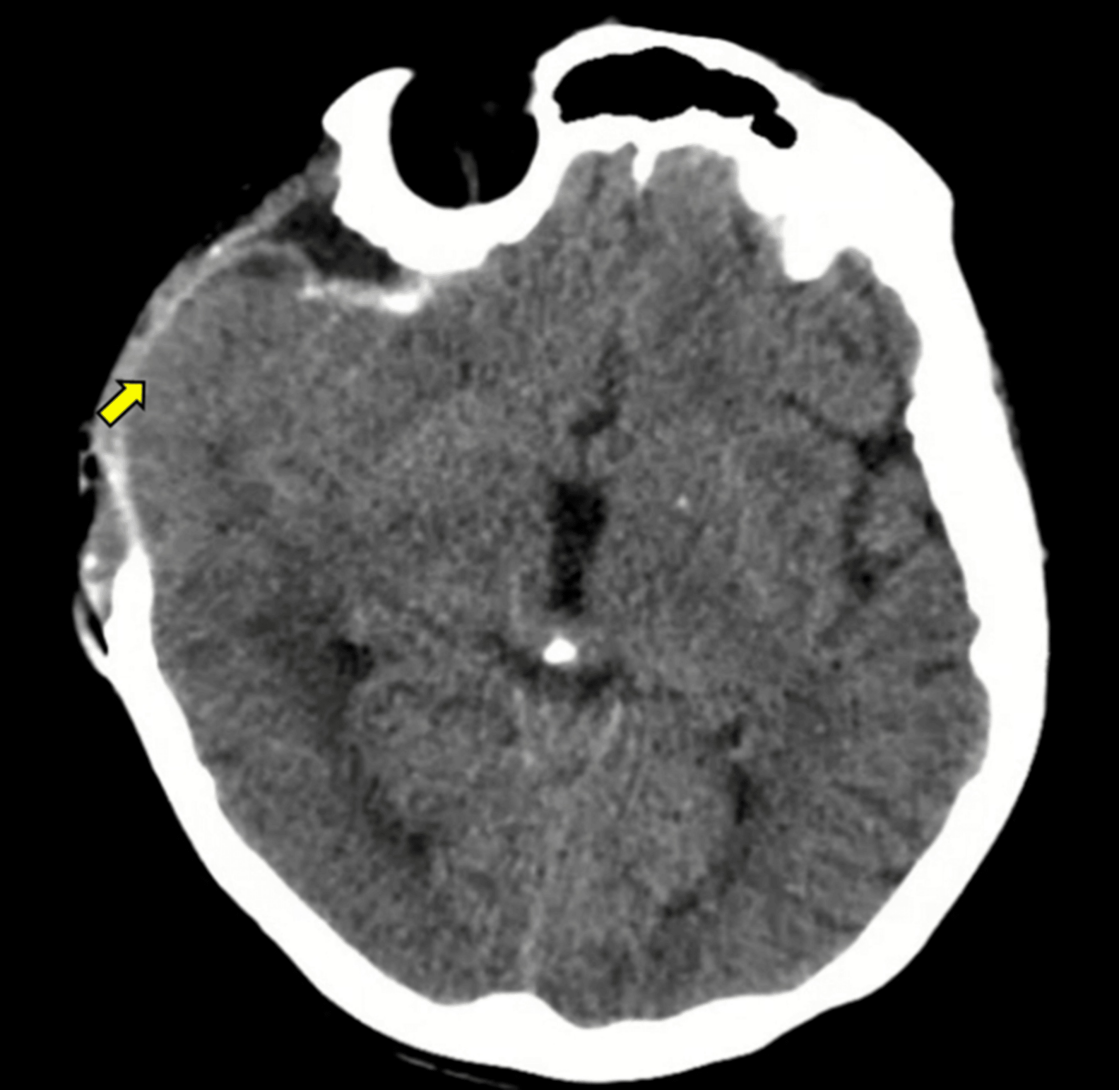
Non-contrast cranial CT scan. Postsurgical changes are observed in the cerebral parenchyma and bone tissue (arrow). No areas of hemorrhage or ischemia are identified CT: computed tomography
